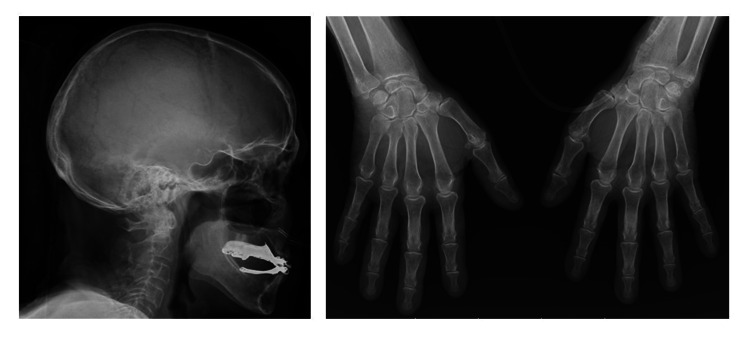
X-ray of the skull (left) and hands (right), without alterations.
